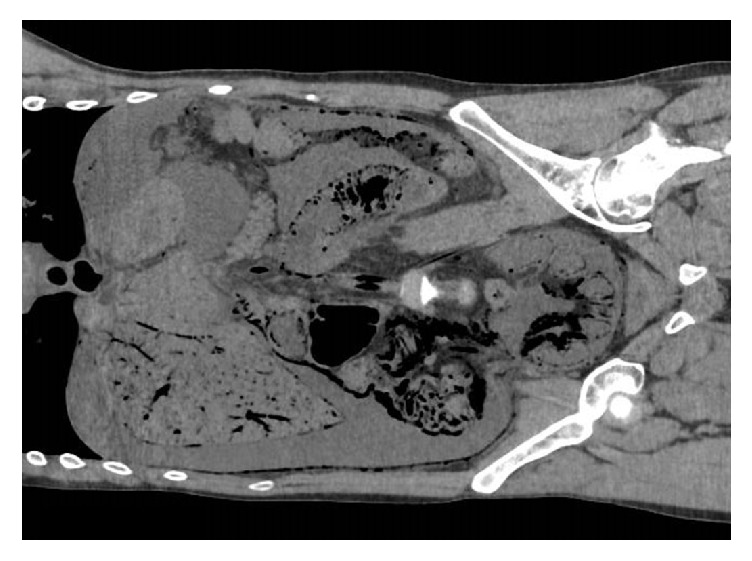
Postmortem CT, coronal view, pneumatosis intestinalis along the small bowel, right colon, and sigmoid, with gas in the portal system and blood vessels, with a large amount of unclear fluid around the liver and in the right paracolic gutter.
